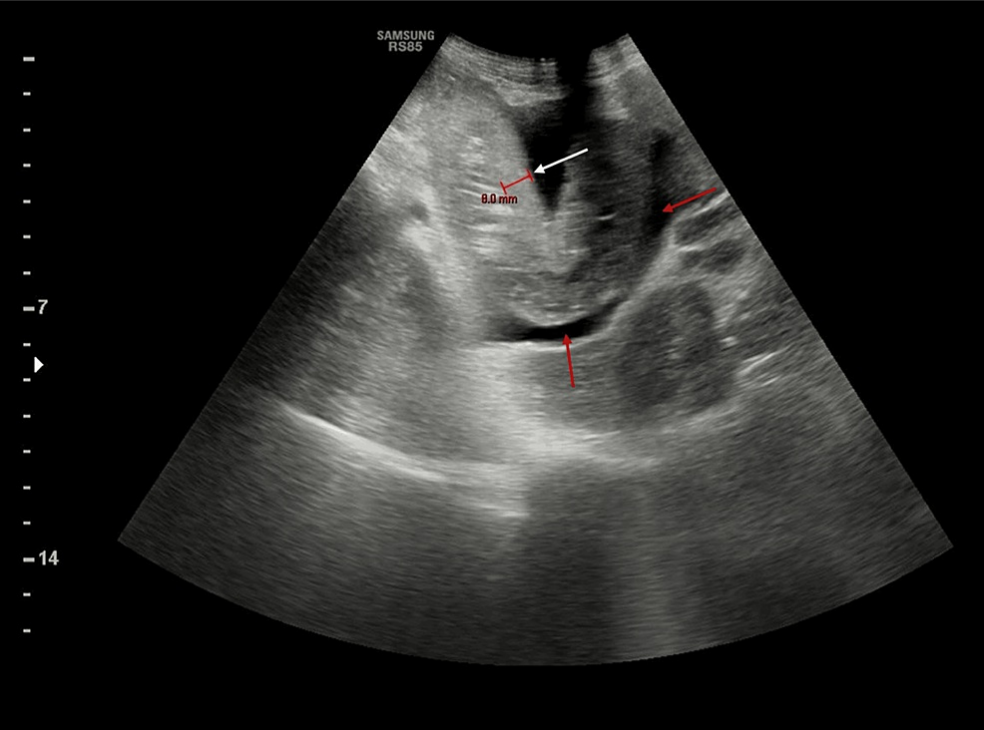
First abdominal ultrasound showing ascites (red arrow) and intestinal wall thickening (white arrow)
